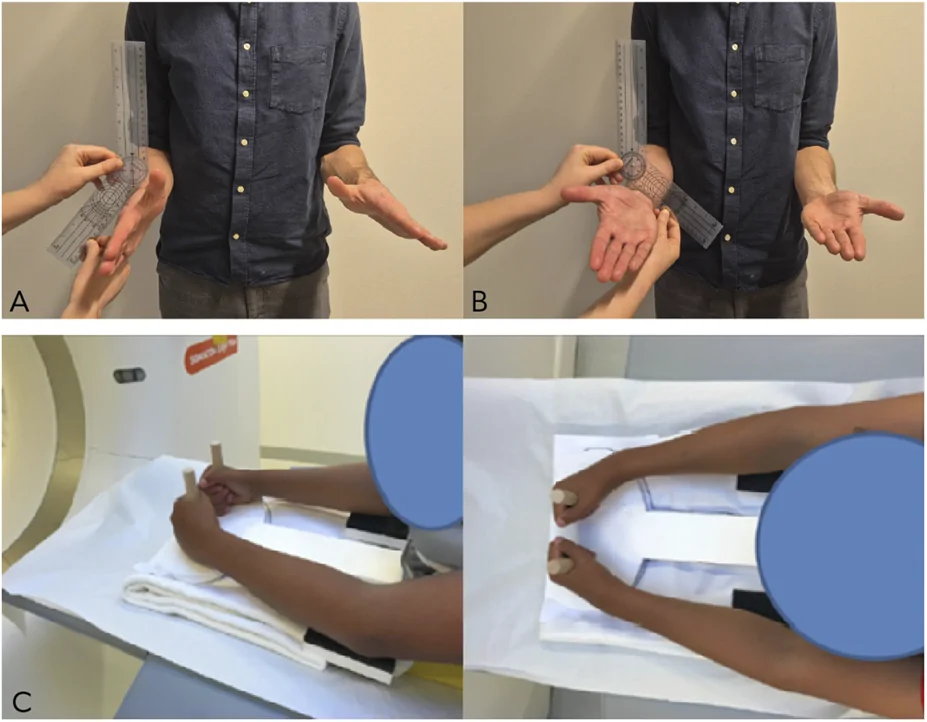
Examples of clinical measurements and scan protocol showing (A) the measurement of pronation and (B) the measurement of supination. (C) Shown here is a patient scanned in a CT scanner in the prone position with the custom holder.
